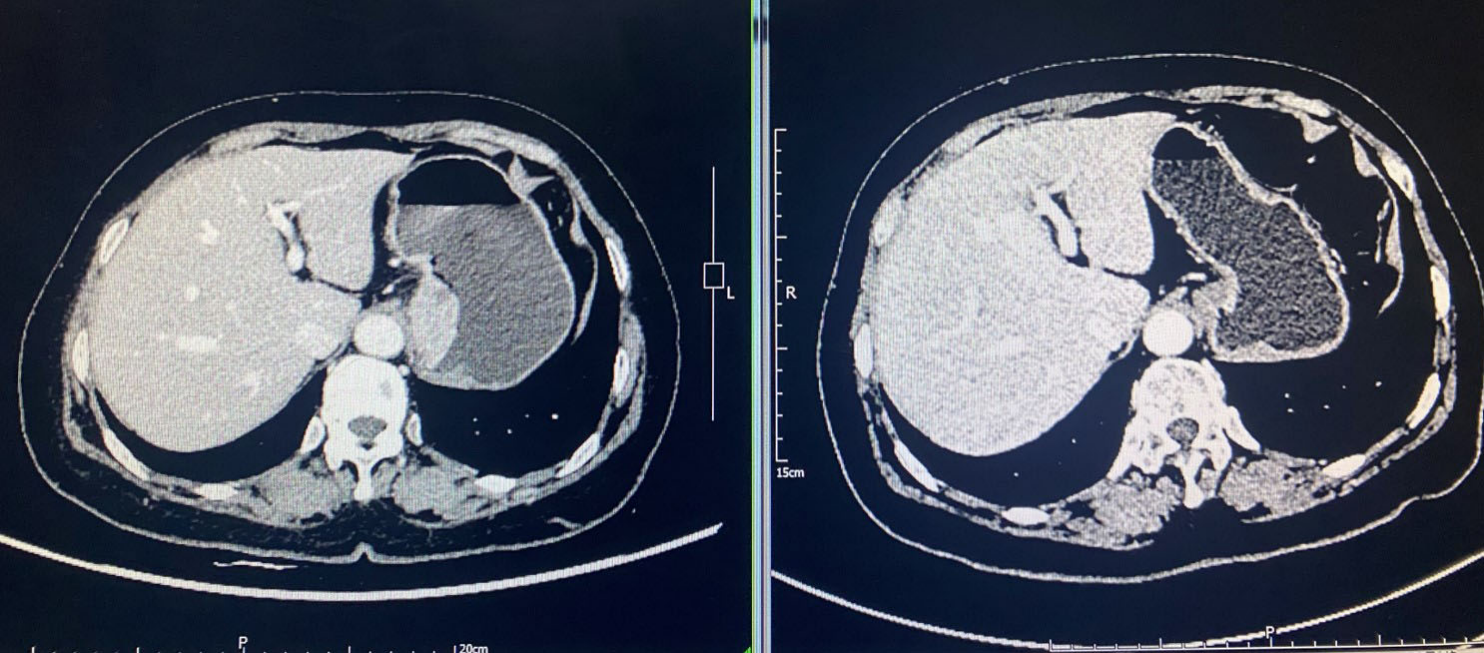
Comparison of abdominal CT scan before and after neoadjuvant therapy. Tumor achieved partial response (PR) with a 50% reduction in the sum of the largest diameters of the tumor target lesions.
